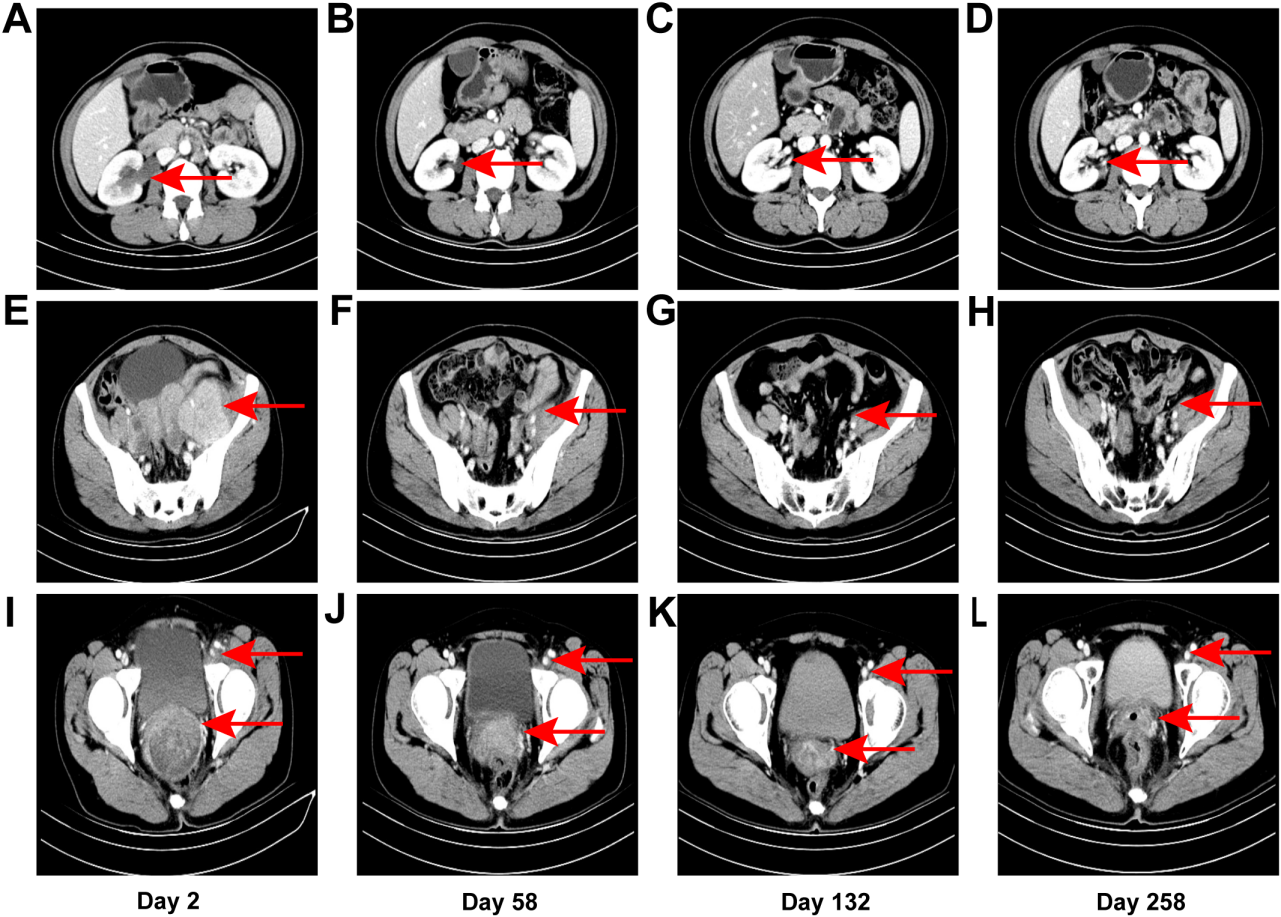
CT images. Right hydronephrosis and right upper ureteral dilation secondary to para-aortic lymph node compression on day 2 from initial contact (A), which was completely relieved on subsequent CT examination [day 58 (B), day 132 (C), day 258 (D)]. Uneven enhancement, pelvic lymph node enlargement with partial fusion on day 2 (E), which exhibited a gradual reduction in size from day 58 (F) to day 132 (G), as well as day 258 (H). Left inguinal lymph node enlargement as denoted by the aforementioned arrows on day 2 (I), which gradually diminished from day 58 (J) to day 132 (K), as well as day 258 (L). Significant thickening of the cervix as indicated by the arrows presented below on day 2 (I), which progressively underwent atrophy from day 58 (J) to day 132 (K), as well as day 258 (L).
